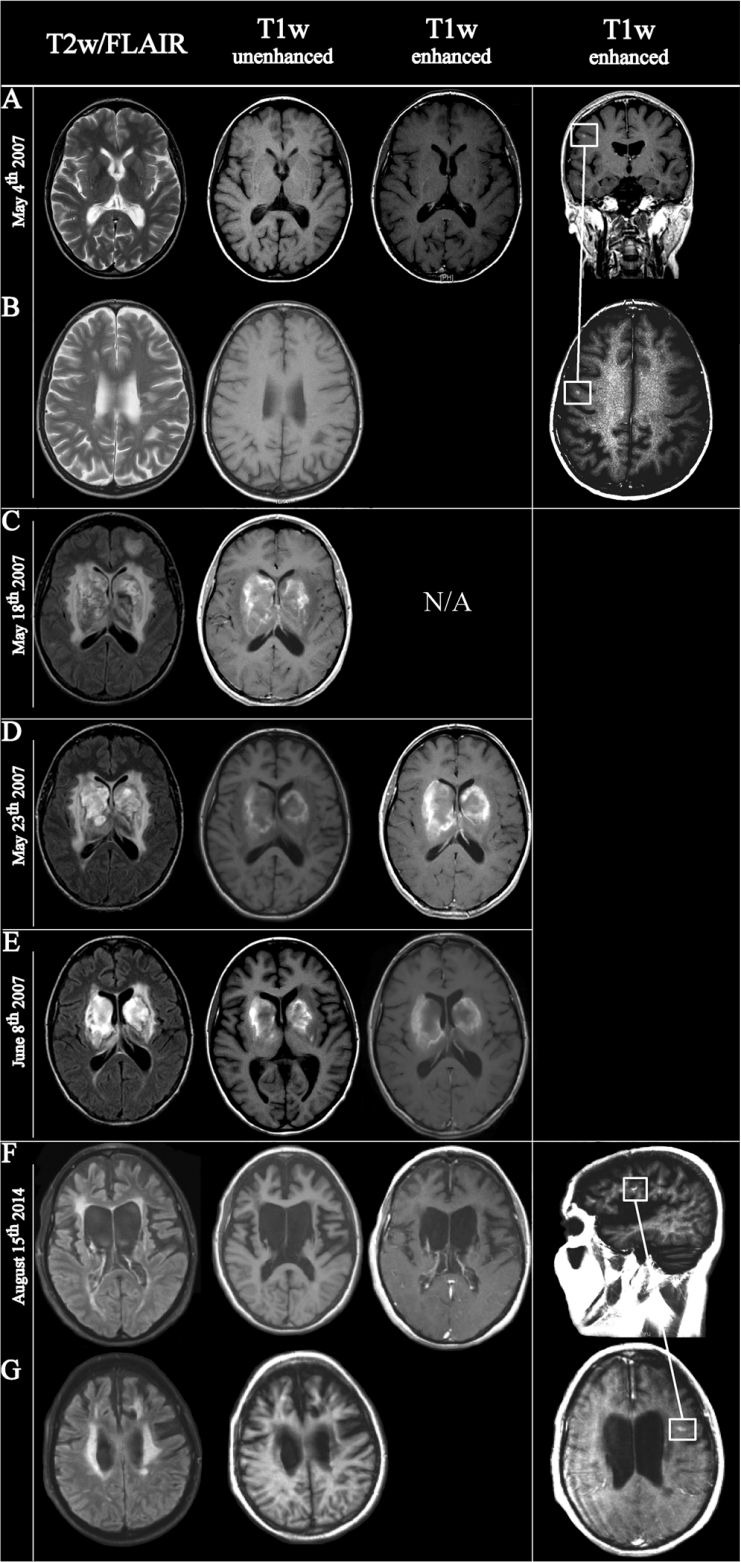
Chronological order of MRI scans from May 4th 2007 until August 15th 2014. a Images show normal deep nuclei and some unspecific small T2-weighted (T2w) hyperintensities. b The cranial T2w sequence depicts the high lesion load accompanied by several black holes in T1-weighted (T1w) sequences. Left-outermost column a–b. The *boxes* indicate a small nodular enhancement in the right precentral gyrus underlining disease progression. c The 2nd exam on May 18th, 2007 reveals the onset of space occupying haemorrhagic changes in the deep nuclei as well as hemorrhagic subcortical foci; hemorrhages are indicated by unenhanced T1w-hyperintensities. d–e The examinations from May 23rd und June 8th show a slow regression of the oedema and the size of the hemorrhagic areas. At both time points and in addition to the T1w signal hyperintensities caused by hemorrhagic residua, contrast enhancements can be found at the outer rim of the lesions. f By August 15th, 2014, only parenchymal defects are remaining together with distinctive brain volume loss leading to enlargement of ventricles. g Axial fluid attenuated inversion recovery imaging (FLAIR) shows an increase in lesions load. Both in the FLAIR and T1w image, confluent and mainly periventricular located lesions are found. Left-outermost column f–g. Boxes indicate a small enhancing focus in the left frontal hemisphere evidencing disease activity in multiple sclerosis. Images from May 5th, 2007 are with courtesy of Röntgenpraxis Georgstrasse, Hannover; images from August 15th, 2014 are with courtesy of Röntgenpraxis Marstall, Hannover. *N/A* not available
